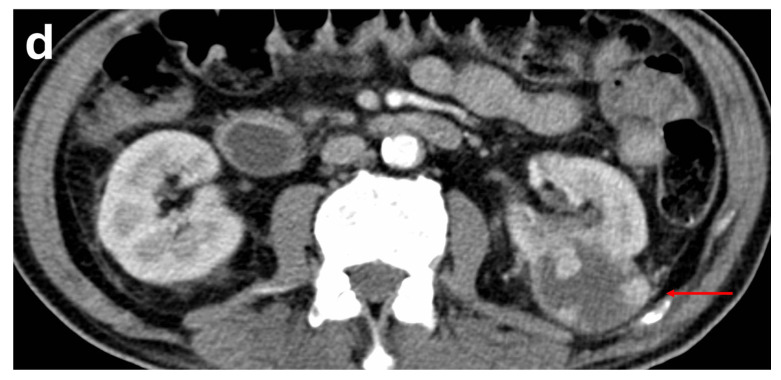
Patient with a CT demonstrating an avidly enhancing, lobulated mass in the left adrenal gland body with central necrosis (red arrows) measuring 4.3 × 3.8 cm. It shows 28 HU on the pre-contrast phase (a), 112 HU on the portal venous phase (b), and 54 HU on the delayed phase (c). Absolute washout was 69.0%, and the relative washout was 51.8%. Imaging through the rest of the abdomen reveals an exophytic solid-cystic mass in the left kidney midpole (red arrow), compatible with a primary renal malignancy (d). Biochemical markers were normal. Surgical resection of the left renal and adrenal masses confirmed the presence of renal cell carcinoma with metastasis of the left adrenal gland.
